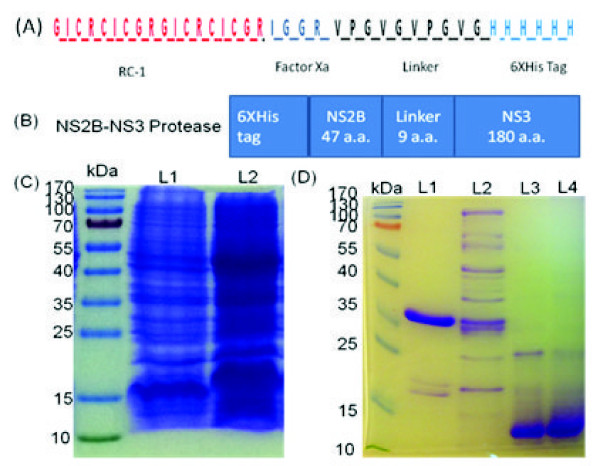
Production of recombinant RC-1 and dengue NS2B-NS3pro by *E. coli. * The sequence of RC-1 was linked by 10 amino acids to a 6XHis tag in pET 303/CT His vector. Factor Xa recognition sequence was inserted to cleave both the linker and 6XHis tag after purification. (A). The NS2B unit of dengue protease was linked to the NS3 by 9 amino acids and the entire DNA fragment was cloned into pQE30 vector downstream of a 6XHis tag (B). The recombinant *E. coli* were cultured in Luria-Bertani liquid medium and induced with Isopropylthio-β-D-galactoside (IPTG) to produce recombinant RC-1 (C, L1 before induction and L2 after induction) and the same procedure was applied to produce recombinant NS2B-NS3pro. The recombinant RC-1 and NS2B-NS3pro were purified by Ni-affinity column (D, L1 purified dengue protease, L2 NS2B-NS3pro before purification, L3 purified RC-1 and L4 purified RC-1 after refolding).
